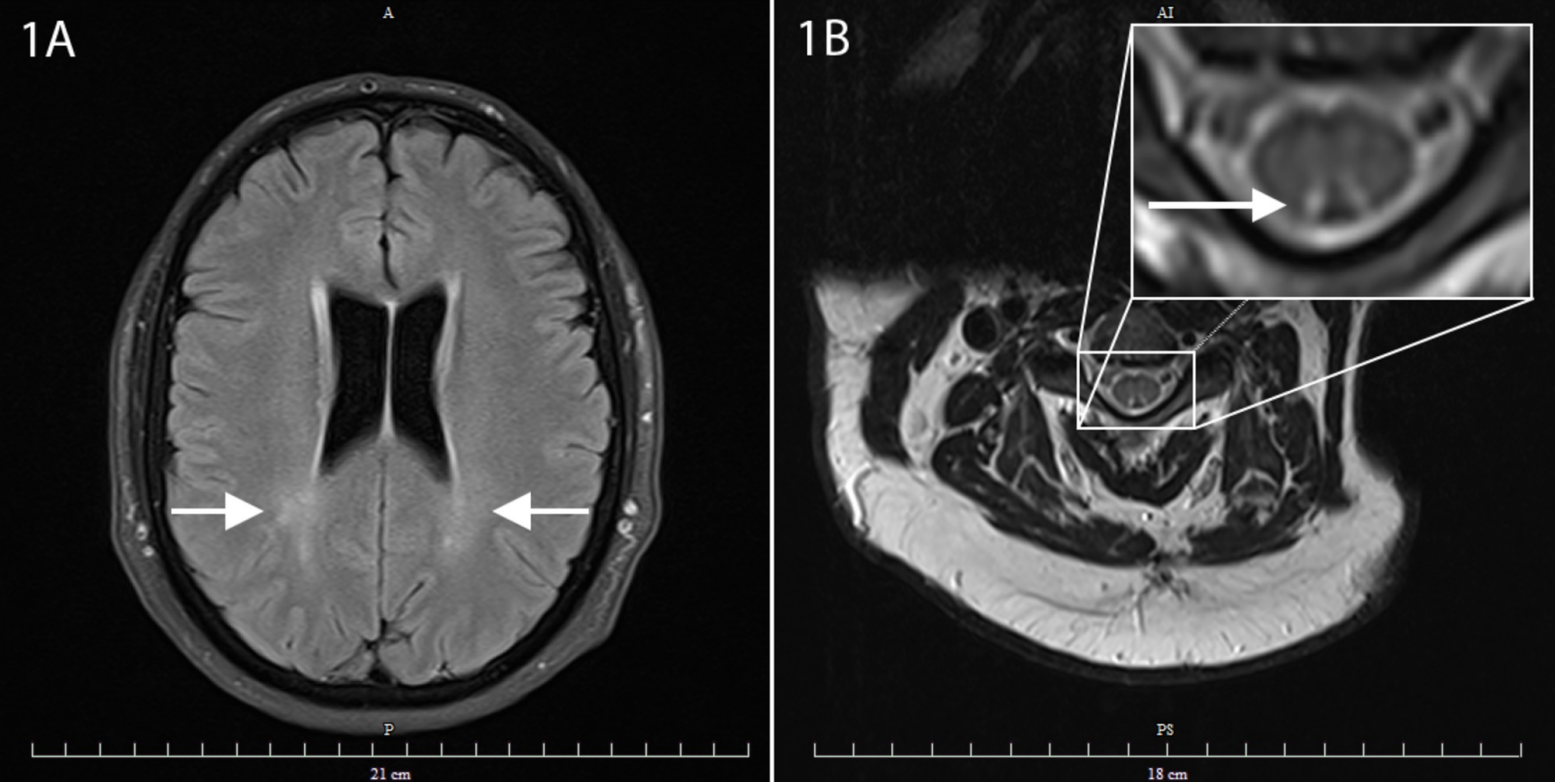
MRI of the neuraxis revealing signs of demyelination A: Axial MRI of the brain showing mild bilateral peri-atrial hazy demyelination without contrast enhancement (white arrows). B: Axial MRI of the cervical spine showing the classic inverted “V” sign suggestive of posterior cord demyelination (white arrow within inset). MRI = magnetic resonance imaging
